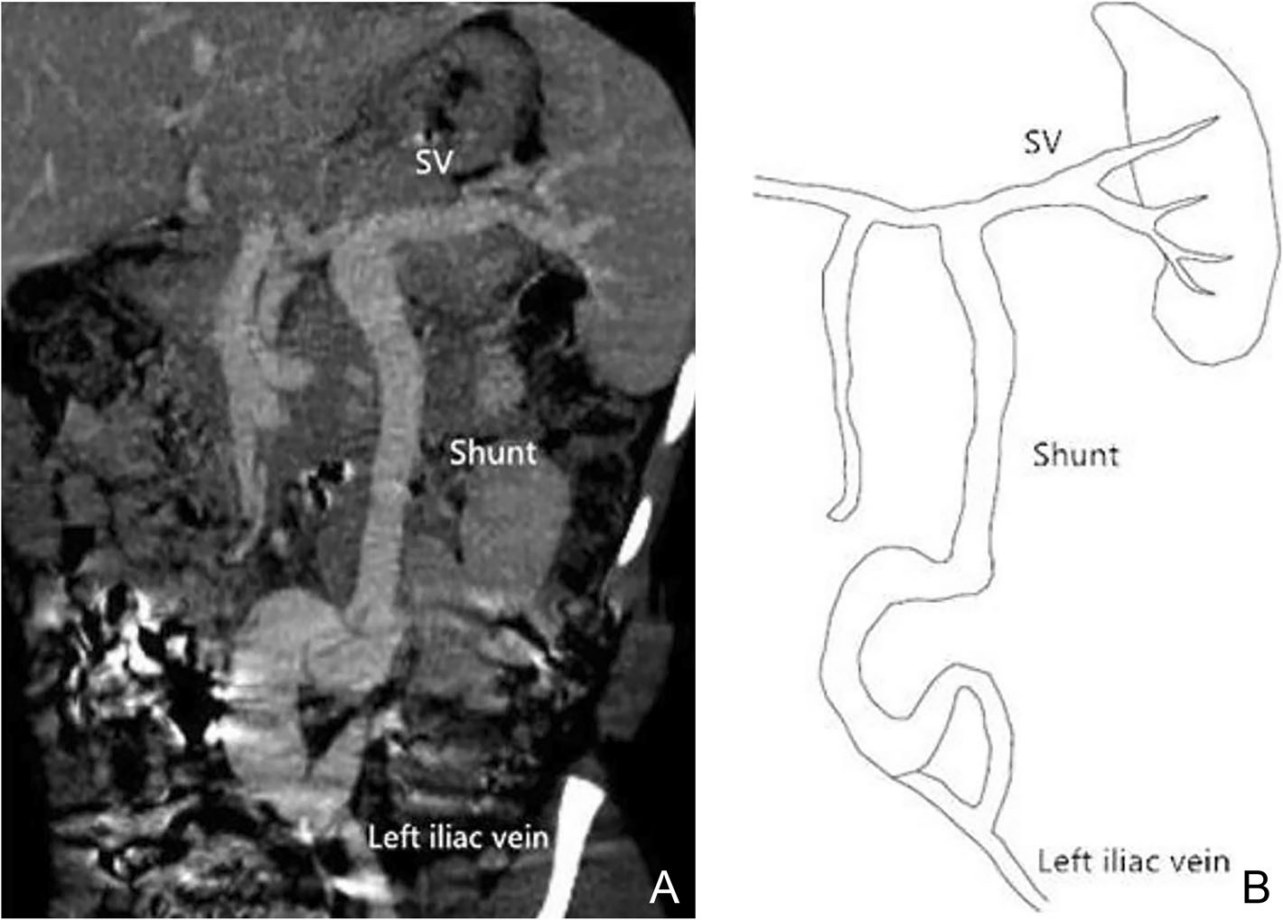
Female, 10 years old, Abernethy II. Abdominal CT angiography maximum intensity projection (MIP) image (portal phase) shows the shunt of extrahepatic portosystemic between splenic vein and left iliac vein
